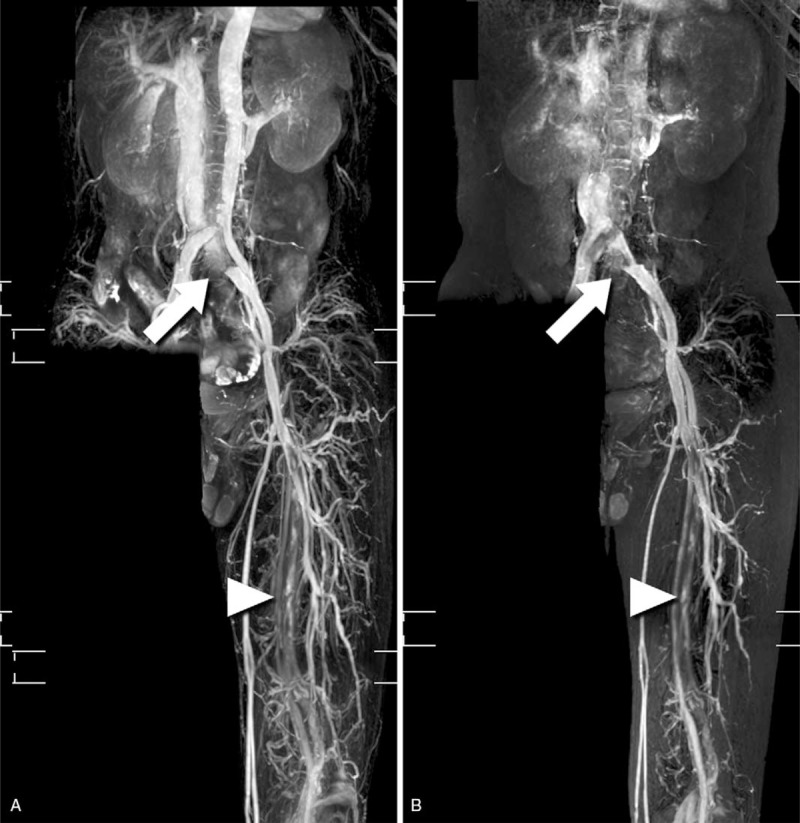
Non-contrast-enhanced magnetic resonance imaging (MRI) using three-dimensional (3D) turbo spin-echo (TSE) with cardiac triggering. (A) For imaging arteries and veins, a 3D-TSE protocol with short tau inversion recovery (STIR) is scanned with diastolic triggering. (B) To obtain veins alone, a 3D-TSE protocol with STIR is scanned with systolic triggering. Sandwich external compression of the common iliac vein (CIV) between the right common iliac artery and lumbar vertebrae (arrow). Flow signal loss of left SFV (arrowhead), indicated infrainguinal deep venous thrombosis.
